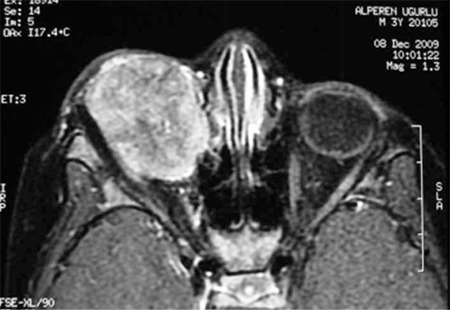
T1-weighted orbital magnetic resonance imaging of retinoblastoma-related buphthalmic bulbus oculi and orbital invasion
